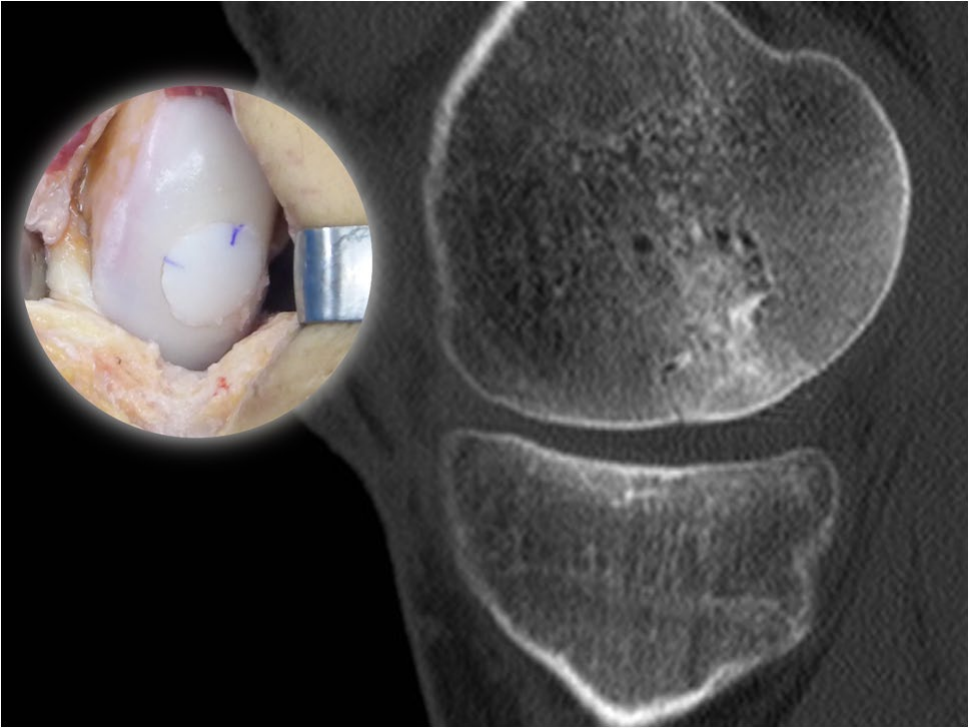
CT scan taken at 6 months and surgical image of a medial femoral condyle FOCA obtaining a low ACTOCA score (1 point)
